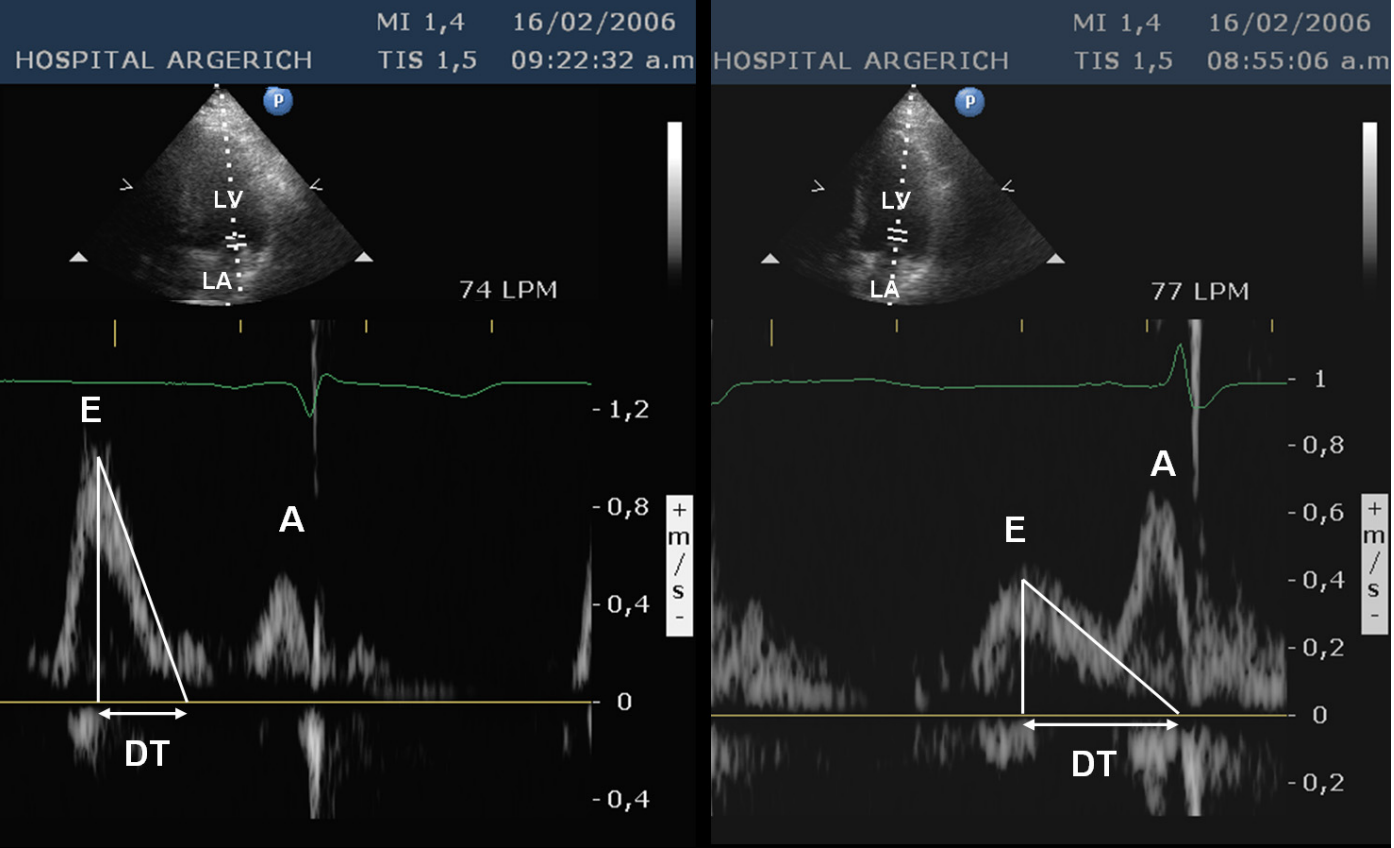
Apical four-chamber view of the left ventricle and Doppler transmitral flow velocity. Left: normal pattern. Right: abnormal pattern of a patient with Chagas' disease. LV: left ventricle, LA: left atrium, E: peak early diastolic velocity, A: peak late diastolic velocity, DT: deceleration time of the early diastolic flow.
